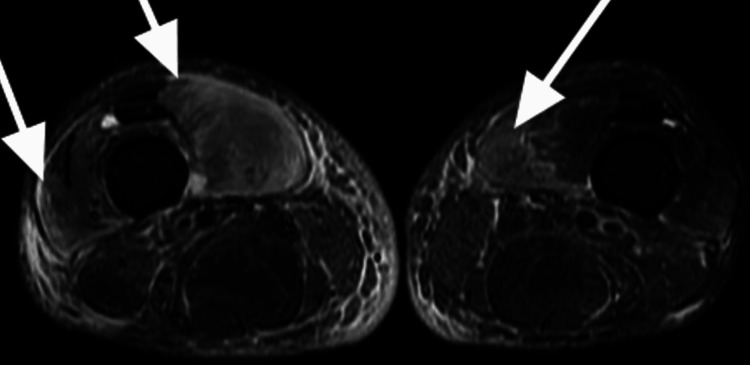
T2-weighted cross-sectional magnetic resonance imaging (MRI) of both thighs showing diffuse edema in the vastus medialis and vastus lateralis muscles on the right thigh, with edema noted in the vastus medialis muscle on the left thigh (white arrows).
